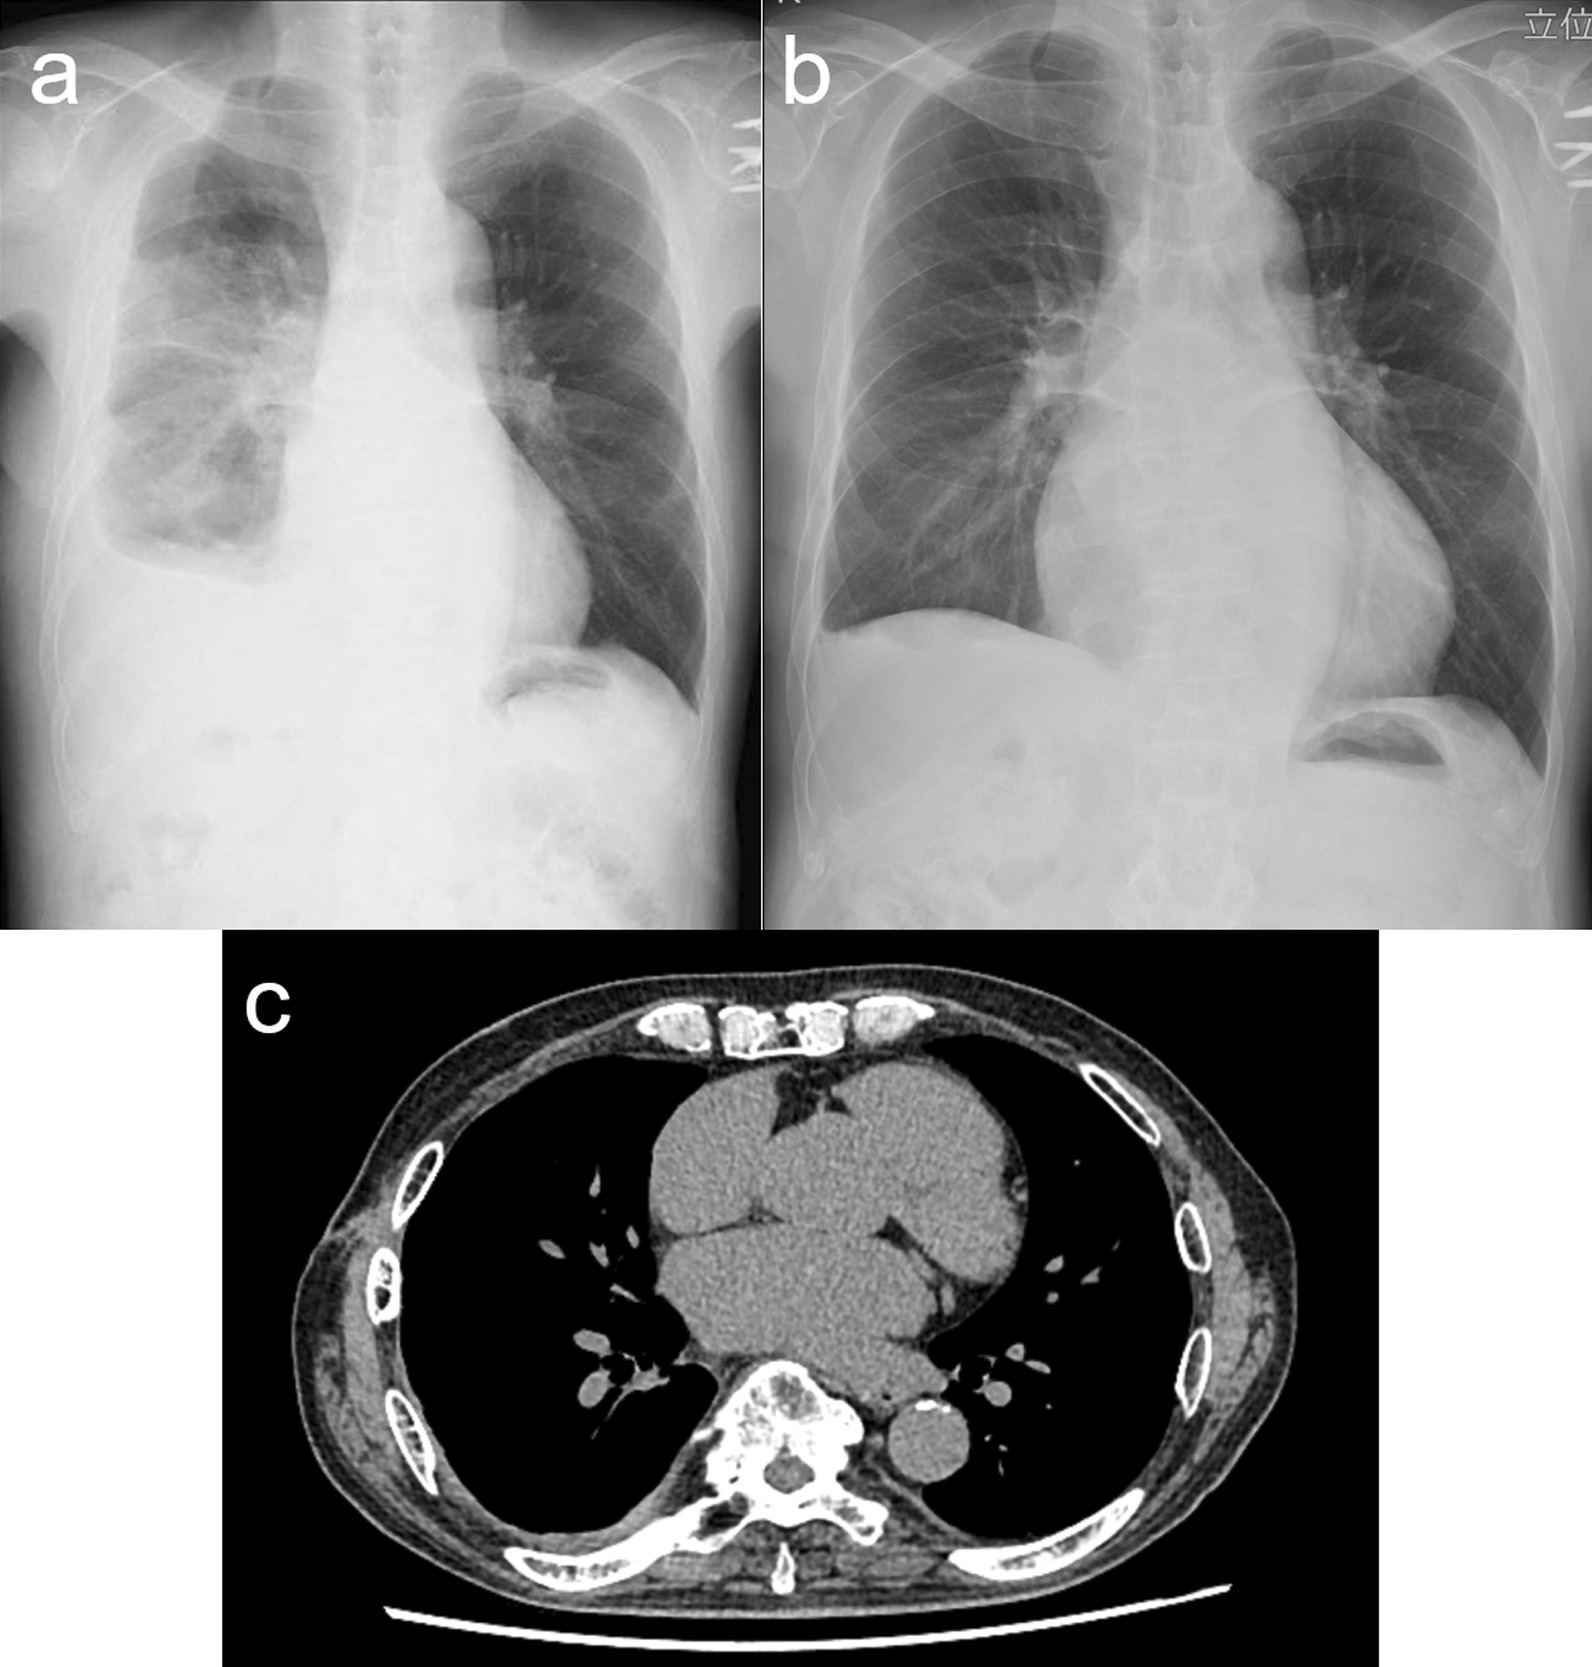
Postoperative images. a Chest X-ray at the discharge. b Chest X-ray and c computed tomography images at the 2-years follow-up examination
